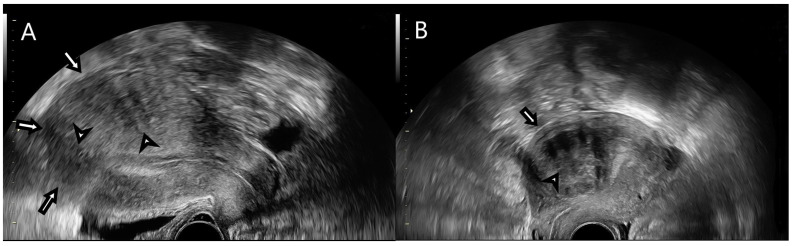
(A) B-mode transvaginal ultrasound features of uterine adenomyosis (highlighted by the arrows): enlarged globular uterus, fan-shaped shadowing and myometrial cysts. (B) B-mode transvaginal ultrasound features of uterine fibroids (highlighted by the arrows): well-defined round lesion within the myometrium with inner fan-shaped shadowing.
